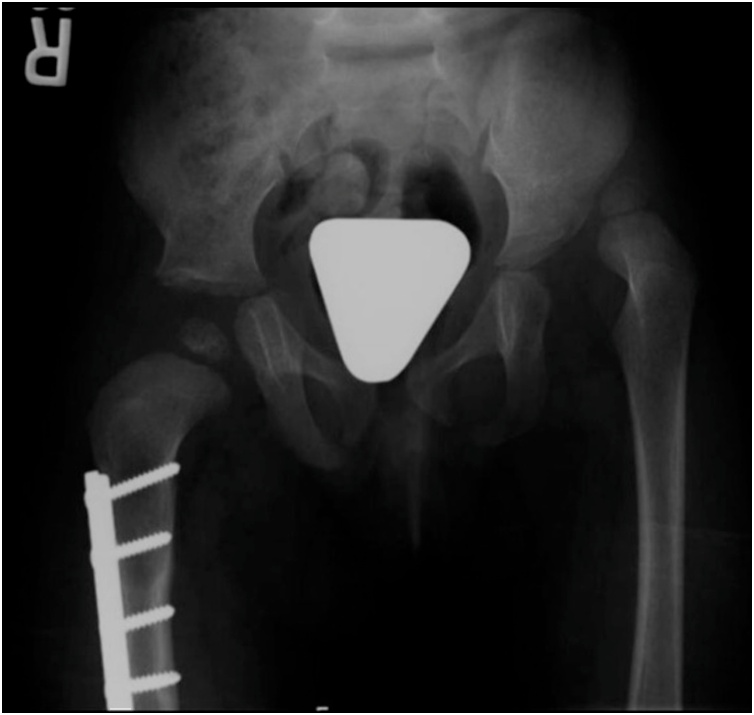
Pelvis AP x-ray at age of 2 ½ years after Right hip open reduction, adductor tenotomy, pelvis osteotomy, femoral shortening and hip Spica.
